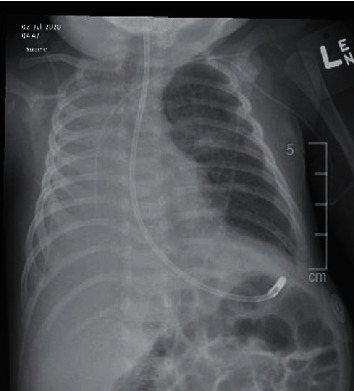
Chest X-ray showing new right lung atelectasis.
